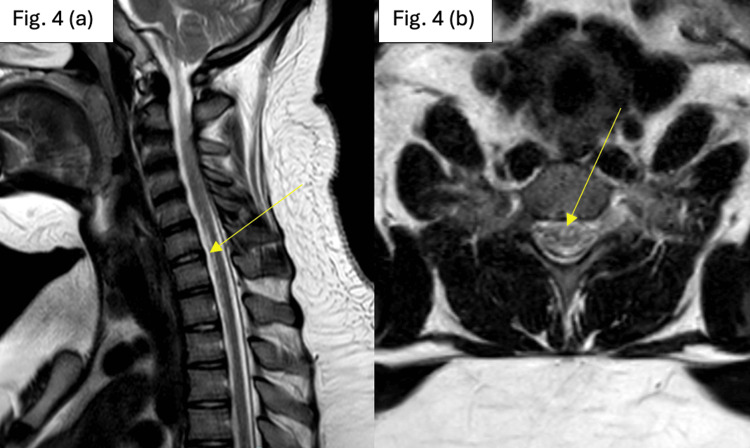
(a) A six-month follow-up sagittal T2-weighted cervical MRI shows high signal intensity from C5 to T3 level (arrow). (b) A six-month follow-up axial T2-weighted cervical MRI at C6 level showed owl’s eye appearance (arrow) of T2 hyperintensity.
